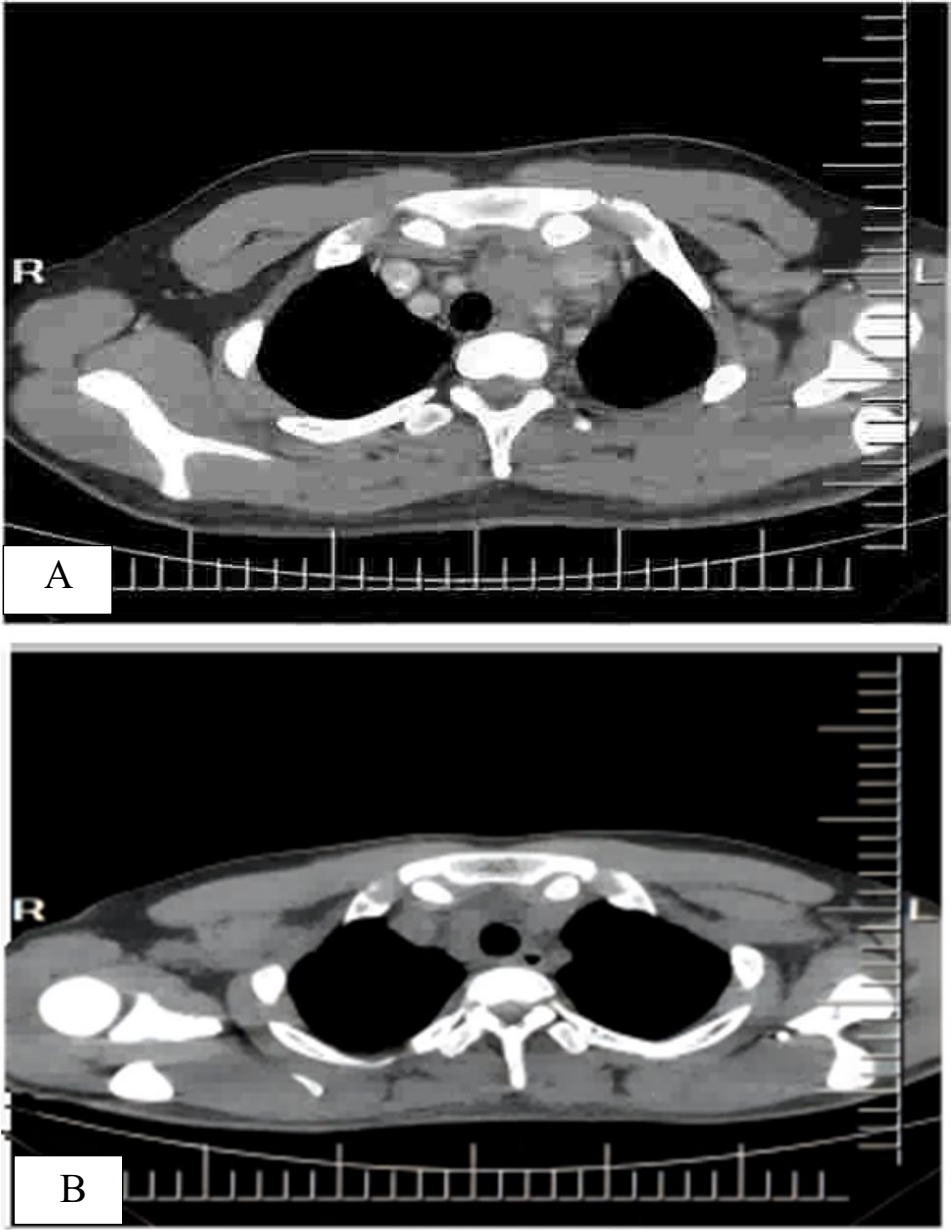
CT scan images are from the same patient (case 5). CT scan showing enlargement or fusion of lymph nodes, with no clear boundary and uniform density or necrosis (A). The follow-up CT scan showed no clear mass and enlarged lymph nodes (B)
